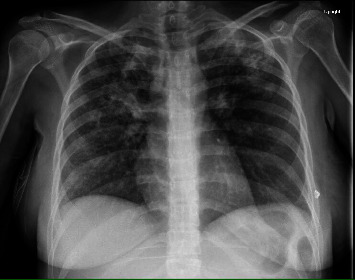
Chest X-ray image showing both alveolar and interstitial infiltrates with findings most prominent within the bilateral upper lobes and right perihilar region.
